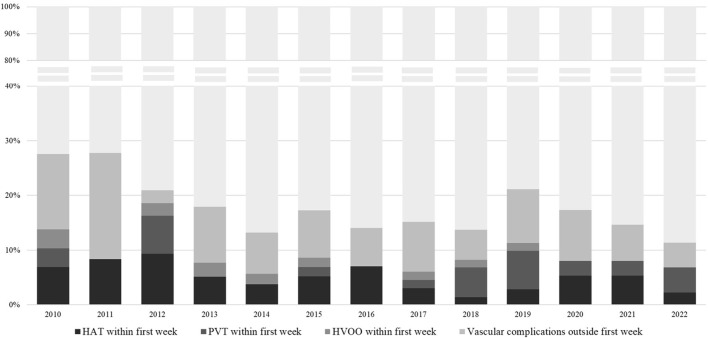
Percentage of vascular complications at any time, HAT within the first week, PVT within the first week and HVOO within the first week, per calendar year of transplantation. rDUS, routine Doppler ultrasound; HAT, hepatic artery thrombosis; PVT, portal vein thrombosis; HVOO, hepatic venous outflow obstruction.
